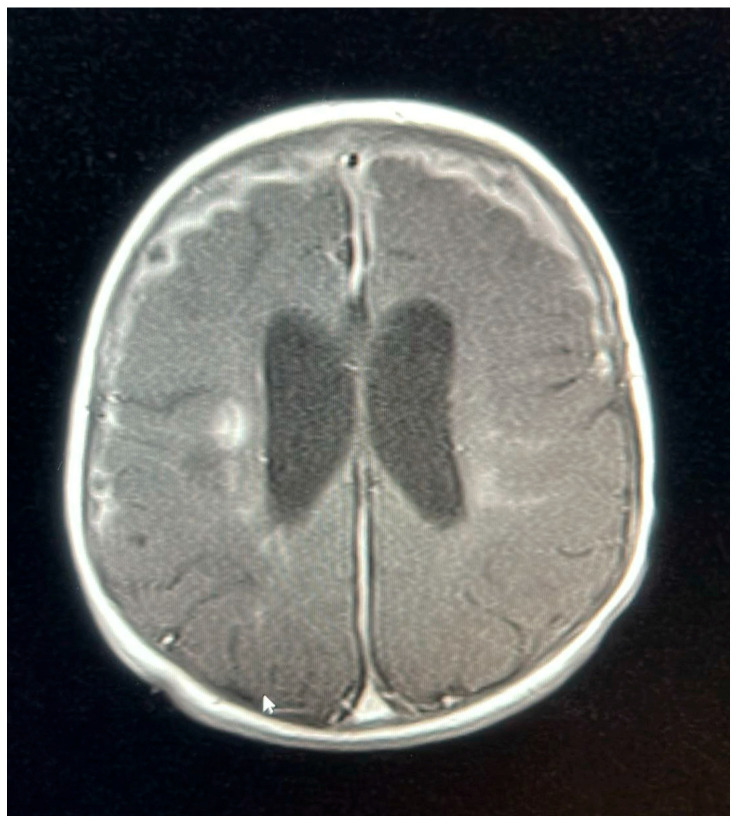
MRI brain scan showingsubdural empyema.
